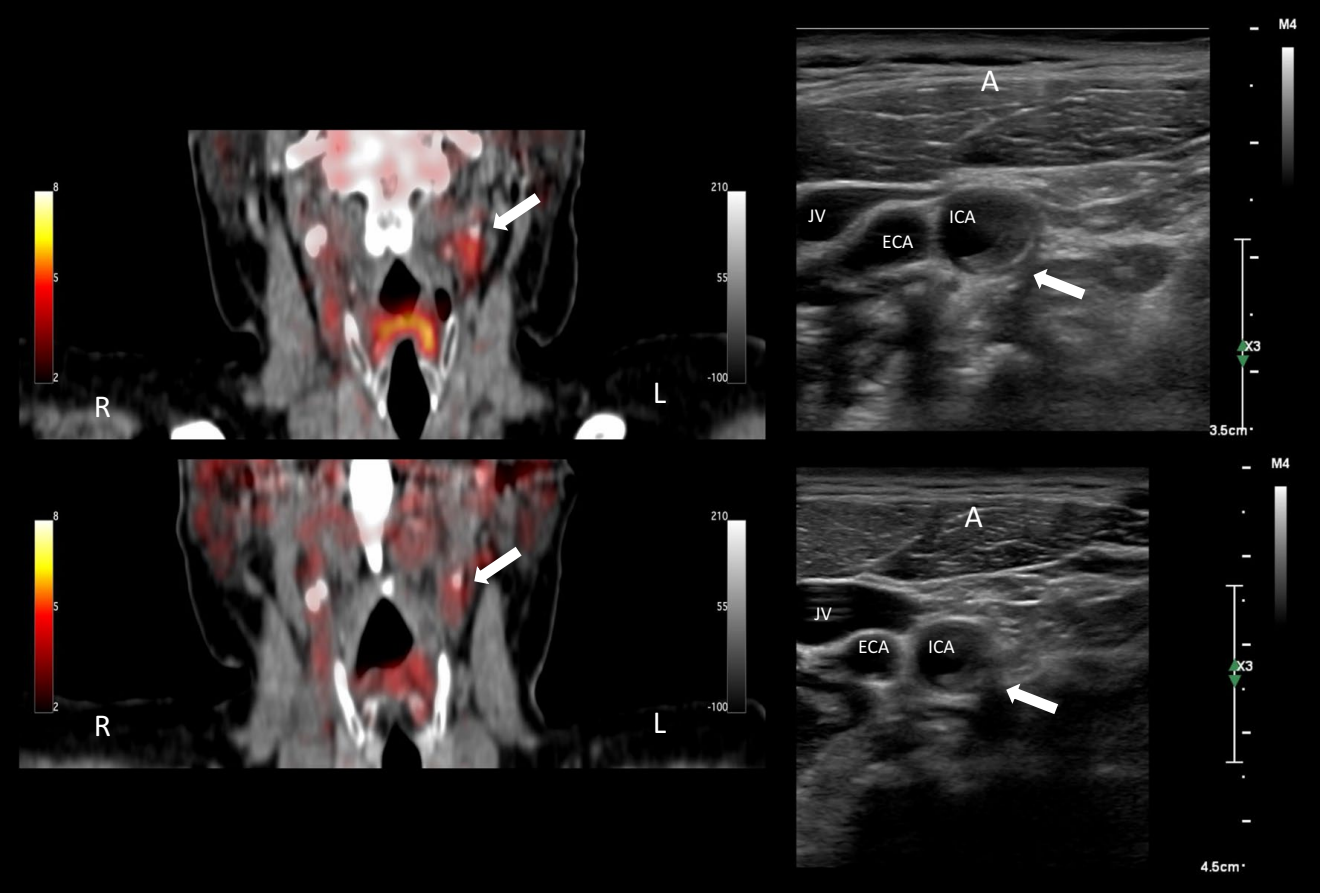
Baseline and follow-up patient example of 2-[18F]FDG-PET / CT-angiography and ultrasound images. Images from one patient. To the left 2-[18F]FDG-PET/CT angiography fusion, frontal MPR and to the right ultrasound images, axial plane. The top images are from baseline and the bottom follow-up. The white arrow points to the plaque on the symptomatic side
